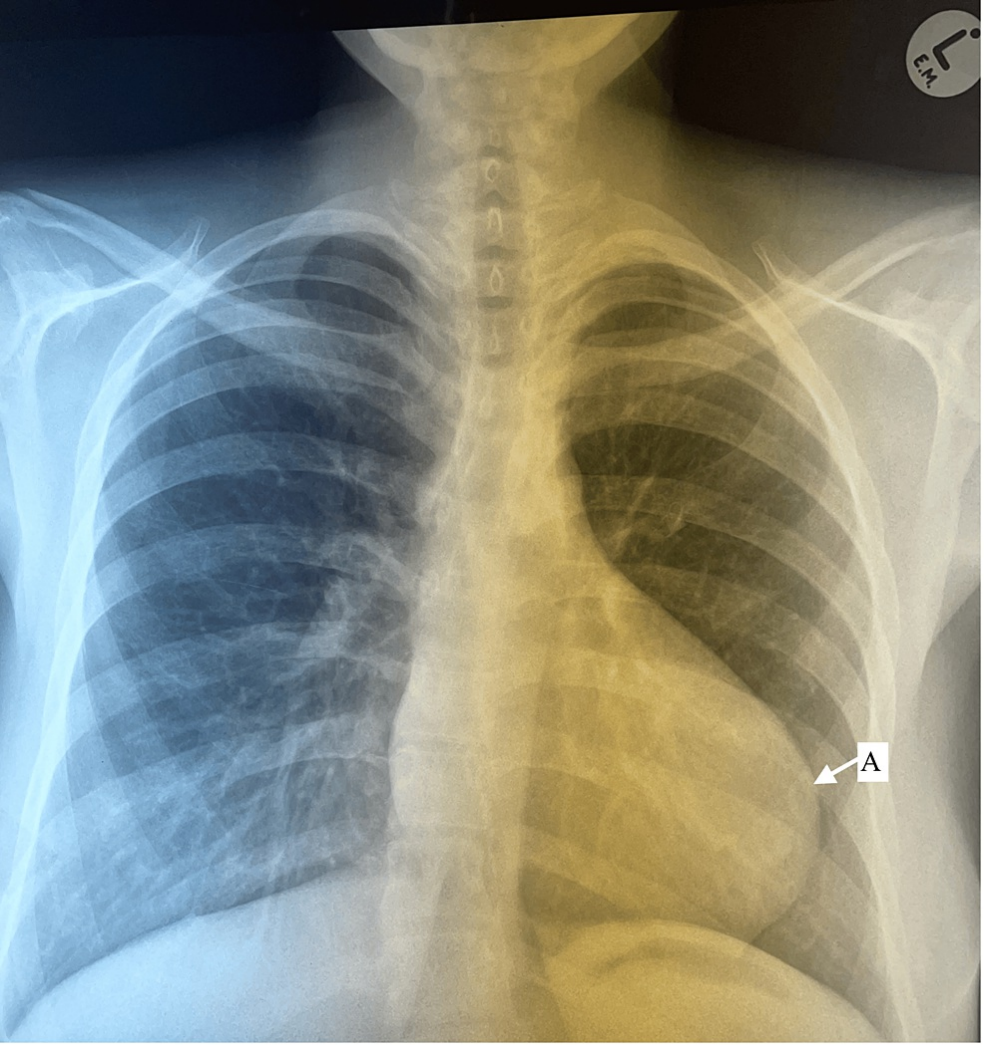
PA Chest X-ray demonstrating the characteristic ‘boot-shaped’ heart (A)
